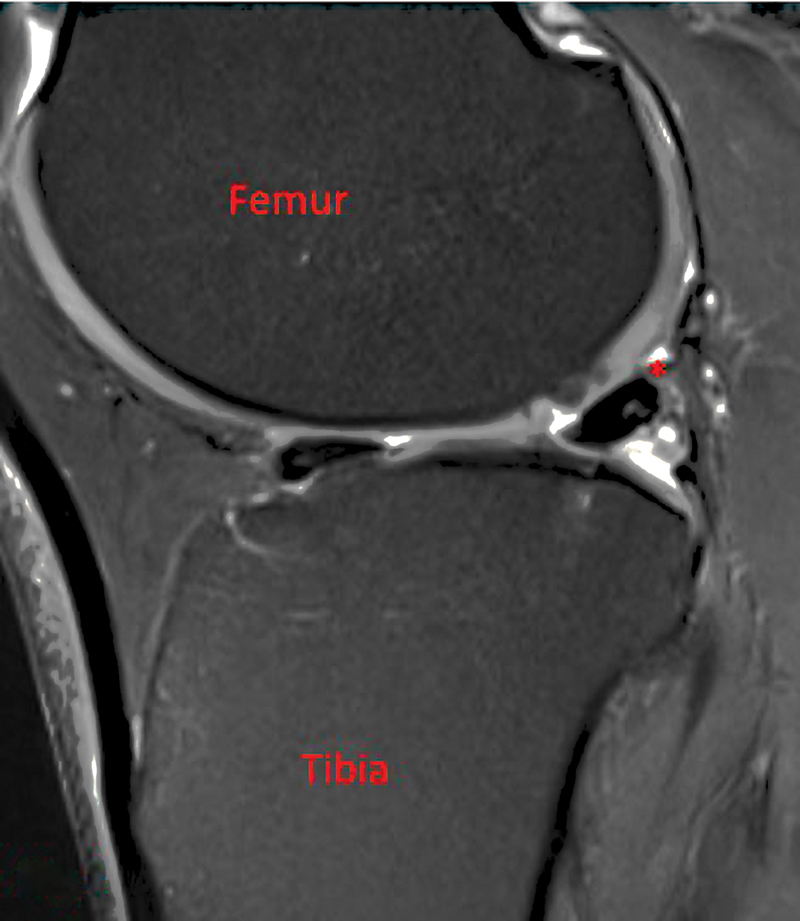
Magnetic resonance imaging showing lateral meniscus tear (*) and flipped fragment (◊).
